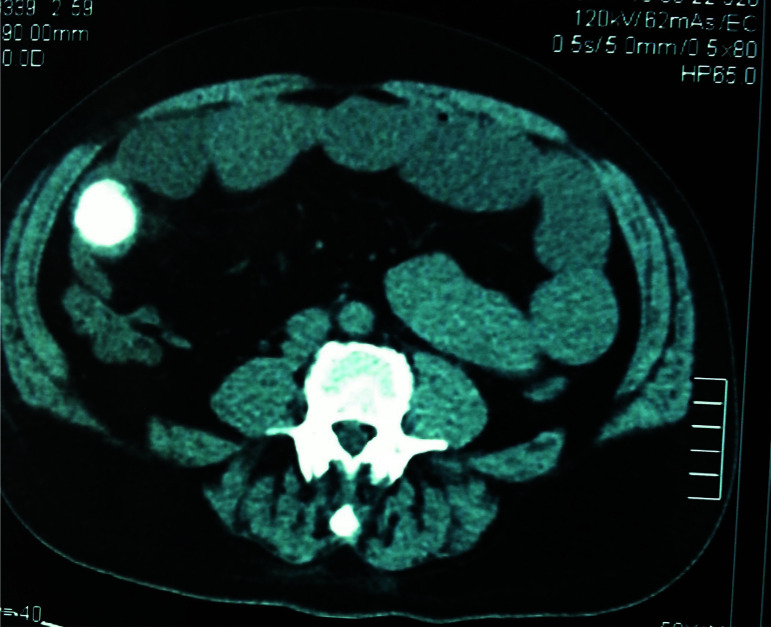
CT scan showing gallstone in the right lower quadrant of the abdomen.
